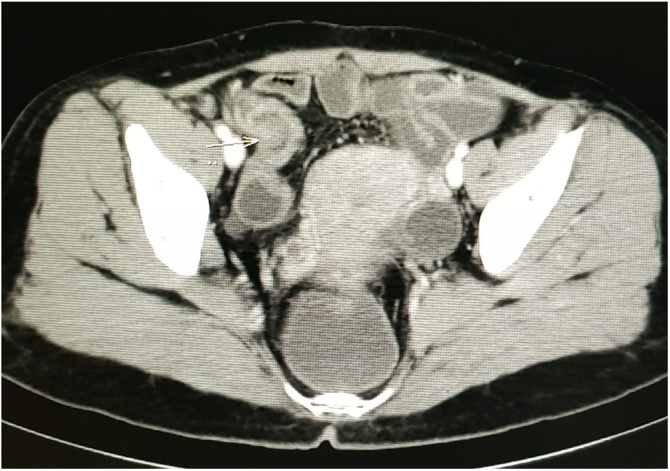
Contrast enhanced computed tomography of abdomen demonstrating a well-defined tubular structure (11 mm in luminal diameter) with lobulated tip (arrow) in relation to the cecum and terminal ileum.
